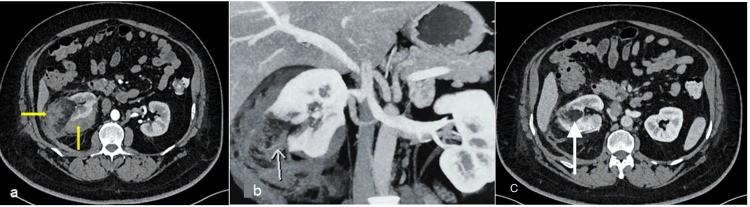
Computed tomography images of the abdomen (a) A subcapsular hematoma in the right kidney with heterodense density lesion seen arising from the interpole of the right kidney (yellow arrows). (b) At 2 weeks, the subcapsular hematoma is seen to be resolving with right renal angiomyolipoma (white arrow). (c) At eight weeks, there is complete resolution of the subcapsular hematoma (white arrow)
